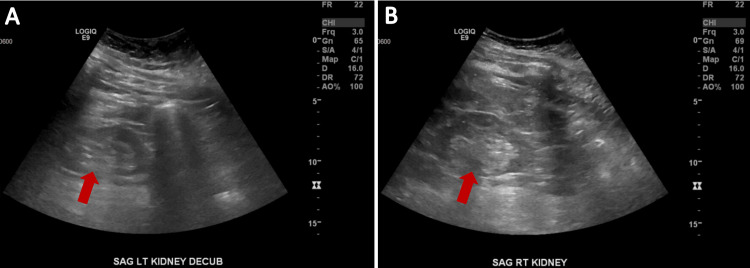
Abdominal ultrasound demonstrating bilateral renal atrophy with diffusely increased echogenicity bilaterally, consistent with CKD. CKD: Chronic kidney disease.
